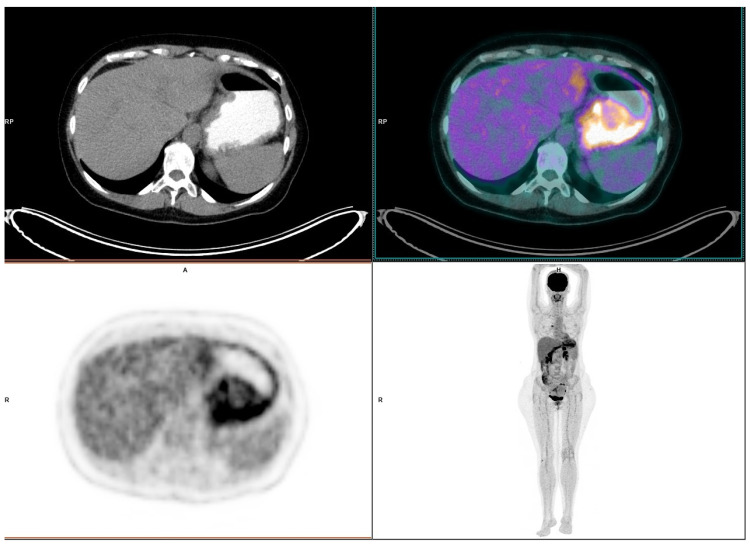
PET/CT, evaluating the metastatic melanoma status revealing intense metabolic uptake in the stomach.
